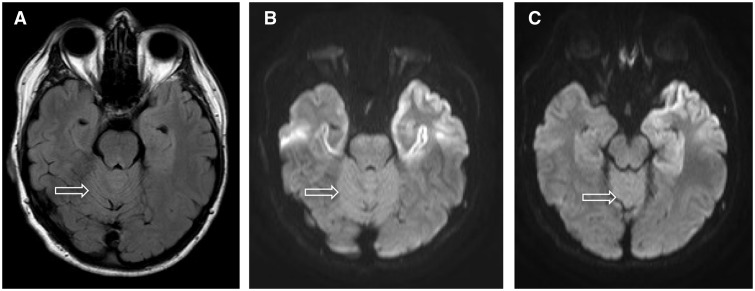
MRI findings in a 47-year-old male patient with iatrogenic CJD due to growth hormone. Hyperintensity in the superior cerebellar vermis is seen on axial FLAIR images (A), and is more conspicuous at DWI at b = 1000s/mm^2^ at the same level (B). (C) A more superior axial image at DWI at b = 1000s/mm^2^ shows the increased vermian signal abnormality compared to the surrounding occipital cortex (Patient 8).
